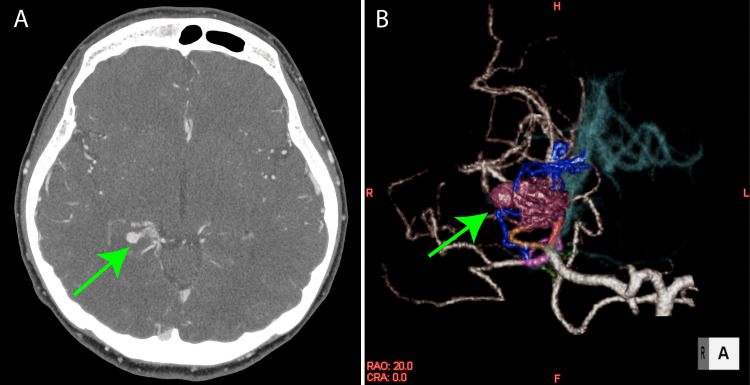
Preoperative images (A) Head-enhanced computed tomography. A horizontal axial view showed arteriovenous malformation at the left middle cerebral artery (green arrow). (B) Volume rendering image revealed arteriovenous malformation at the left middle cerebral artery (green arrow).
